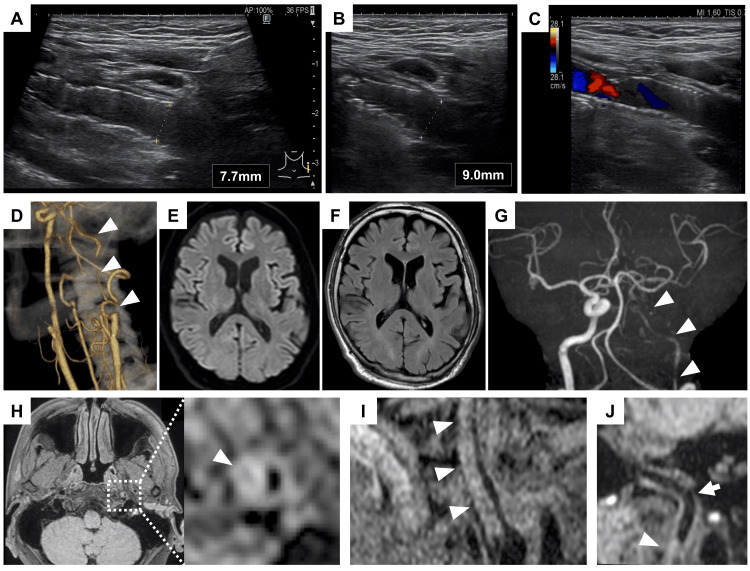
Imaging findings left ICA dissection A-B: Carotid ultrasound on postoperative day 5 showing dilation of the distal stent segment and the ICA beyond the stent; C: Color Doppler ultrasound of the neck on postoperative day 5 showing absence of blood flow in the ICA distal to the stent; D: Neck CTA on postoperative day 5 shows poor visualization of the ICA distal to the stent. Arrowheads indicate the ICA with reduced visibility; E-F: DWI and FLAIR on postoperative day 5, showing no high-intensity lesions in the left cerebral hemisphere; G: Head MRA showing poor visualization of the left ICA; H-J: 3D-T1-VISTA reveals a crescent-shaped deformation of the arterial lumen due to the dissection flap extending from the distal stent region to the proximal petrous portion of the left ICA. Arrowheads indicate the dissection flap, and the arrow indicates the petrous portion of the left ICA. CTA: computed tomography angiography; DWI: diffusion-weighted imaging; ICA: internal carotid artery; FLAIR: fluid-attenuated inversion recovery; MRA, magnetic resonance angiography, 3D-T1-VISTA: Three-dimensional T1-weighted volume isotropic turbo spin-echo acquisition
